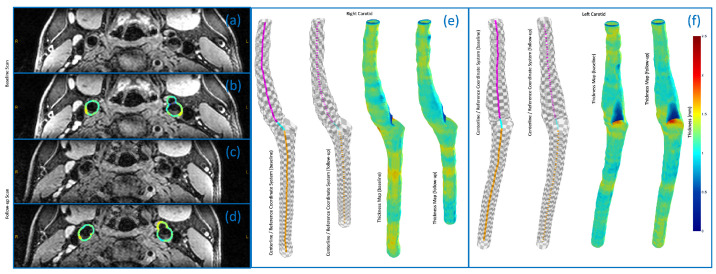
(a,c) Axial views of 3D MERGE MR imaging data for the baseline and follow-up scans. (b,d) The corresponding vessel wall thickness maps superimposed on the axial views. (e,f) Extracted centerlines, bifurcation coordinate systems, and vessel wall thickness maps on the luminal surfaces in baseline and follow-up scans for the right (e) and left (f) carotid arteries.
